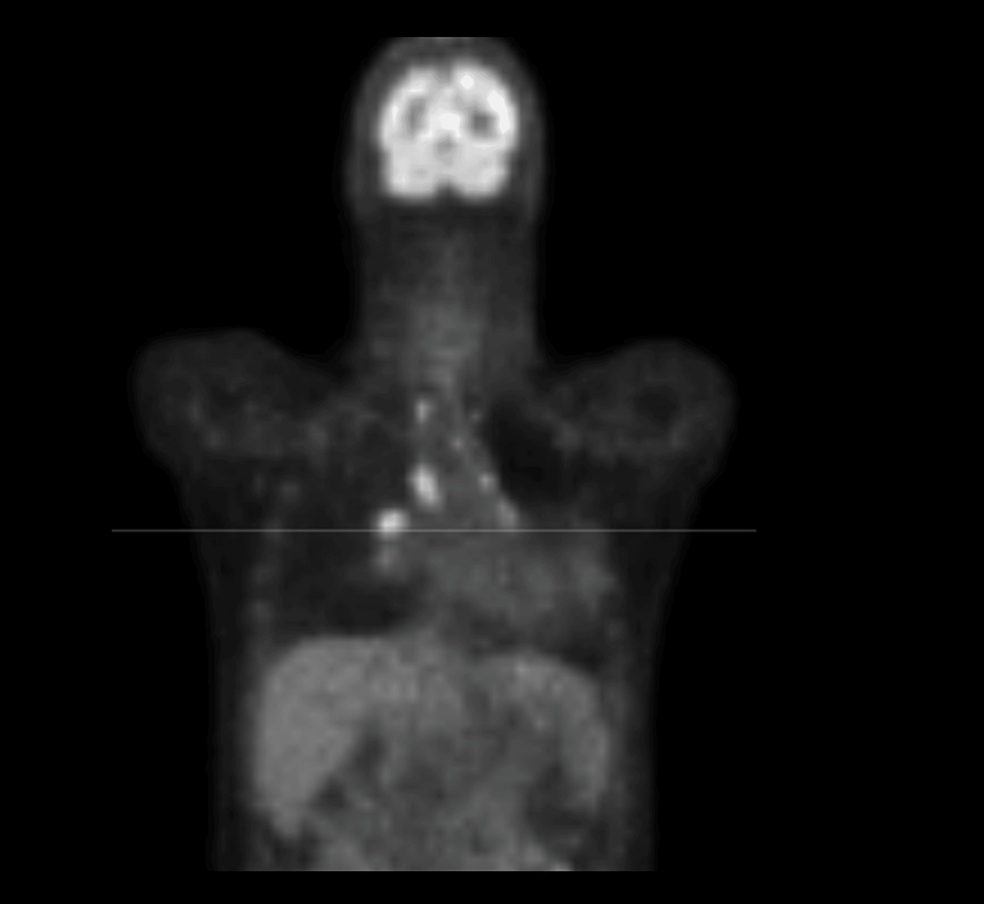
FDG-PET showing left bronchogenic carcinoma and contralateral hypermetabolic hilar adenopathy and upper mediastinal lymph nodes (October 26, 2011) FDG-PET: fluorodeoxyglucose-positron emission tomography
